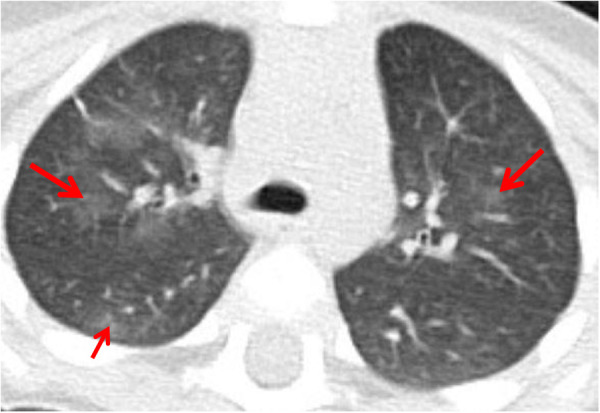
Chest CT findings in a 24-month-old female with acute exogenous lipoid pneumonia following accidental sewing machine oil ingestion.the patient presented to the hospital 12 h after exposure with fever and shortness of breath.Chest CT (lung window) shows patchy consolidation and ground glass opacities (arrows) in both lower lobes.
